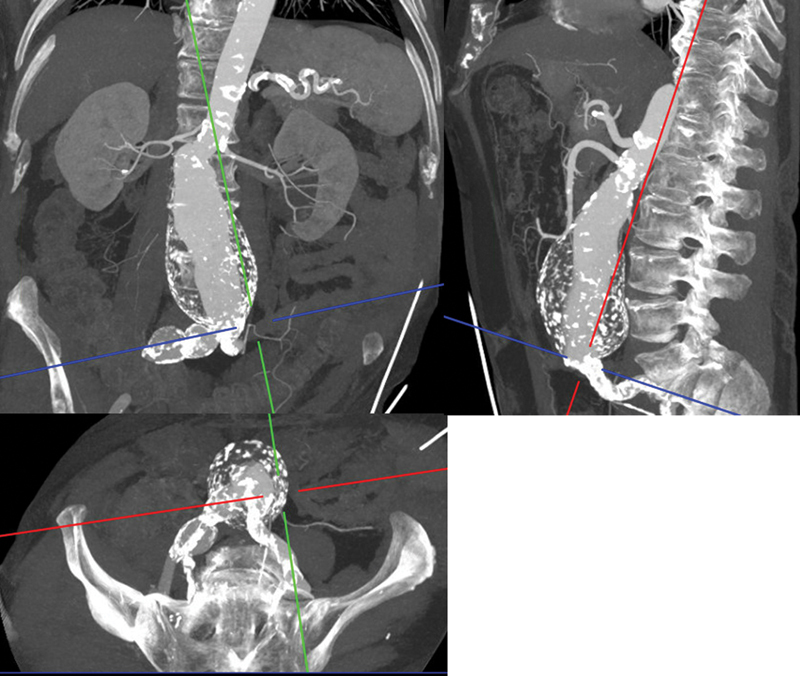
Multiplanar reconstructions of the preoperative CTA scan showing the large juxtarenal abdominal aortic aneurysm (AAA) and right common iliac artery aneurysm with subtotal occlusion of the right internal iliac artery. CTA, computed tomography angiography.
